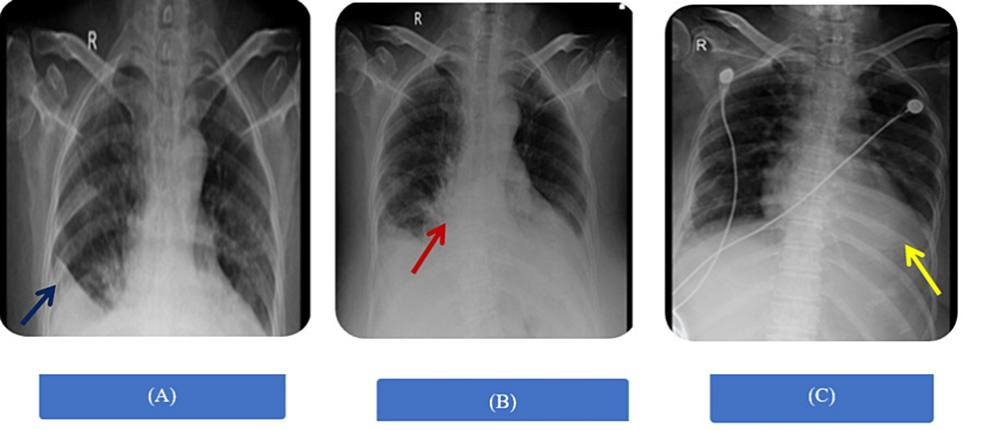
The patient's chest X-ray showed: (A) Homogenous opacities seen on bilateral lower zones with obliterated costophrenic angle on the right side; (B) Homogenous opacities seen on the right side with the presence of air bronchogram; (C) Obliteration of left costophrenic angle with diffusely visible cardiac margins on the ipsilateral side.
